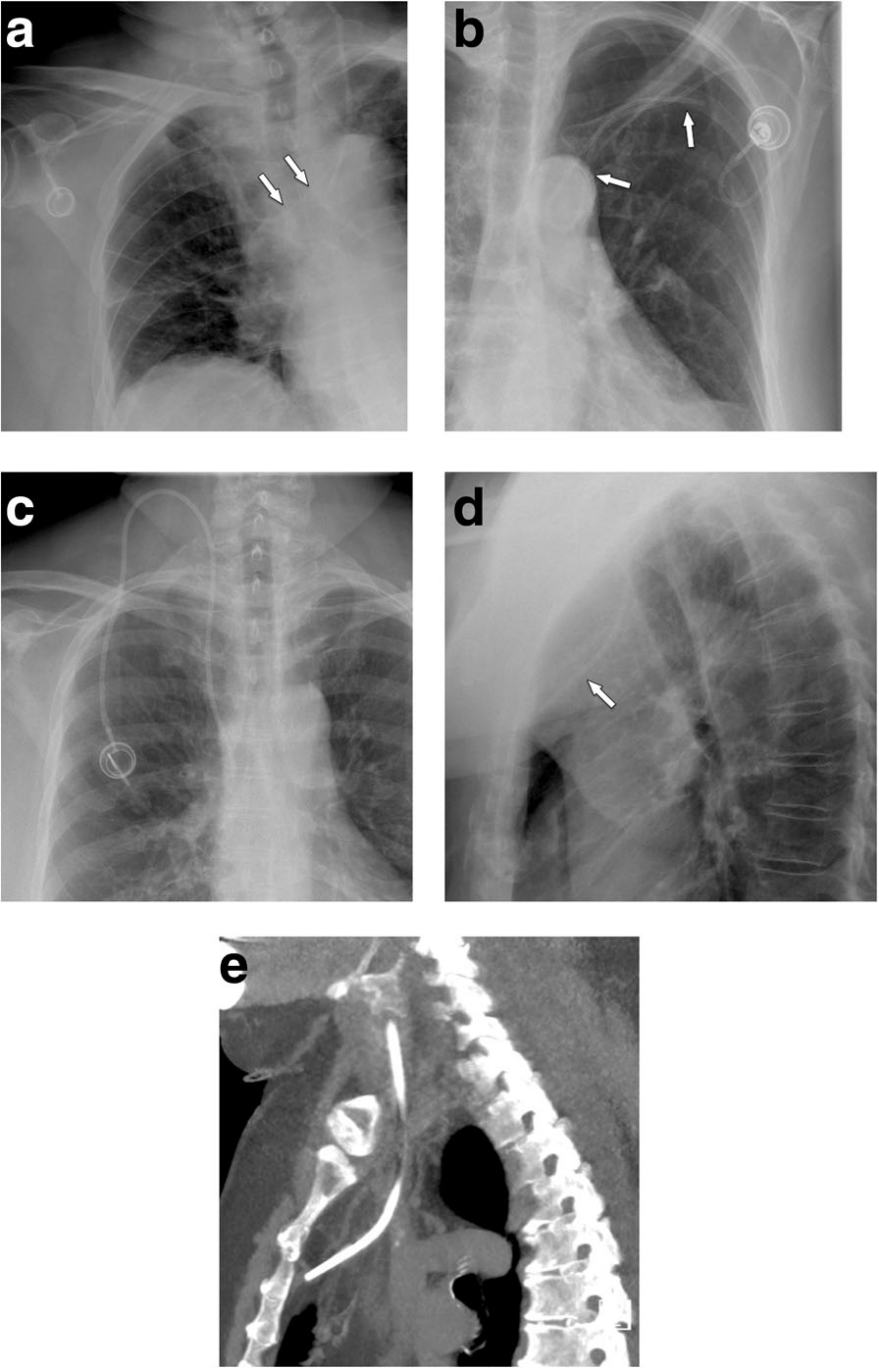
Common venous malpositioning. a The venous catheter, implanted via the subclavian vein, enters the contralateral brachiocephalic vein (arrow). b Loop formation in the subclavian vein. c–e Malpositioning in the mammarian vein due to occlusion of the SVC. c PA chest film shows normal course of the catheter, which had been implanted into the internal jugular vein. d However, the lateral projection shows an abnormal anterior course. e Sagittal MIP CT reconstruction confirms malpositioning into the internal mammary vein
